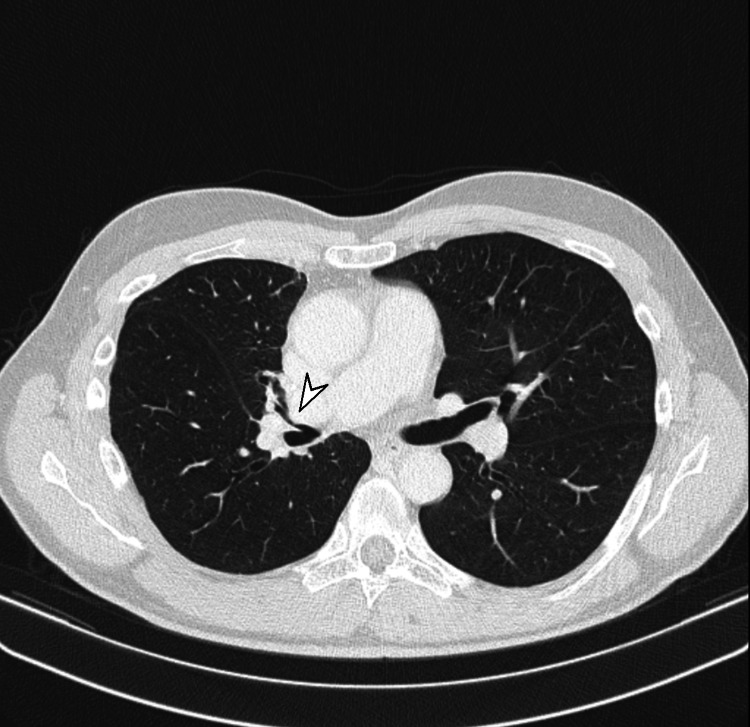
Chest CT showing an upper right lobar bronchus stenosis (white arrowhead) CT: computed tomography
